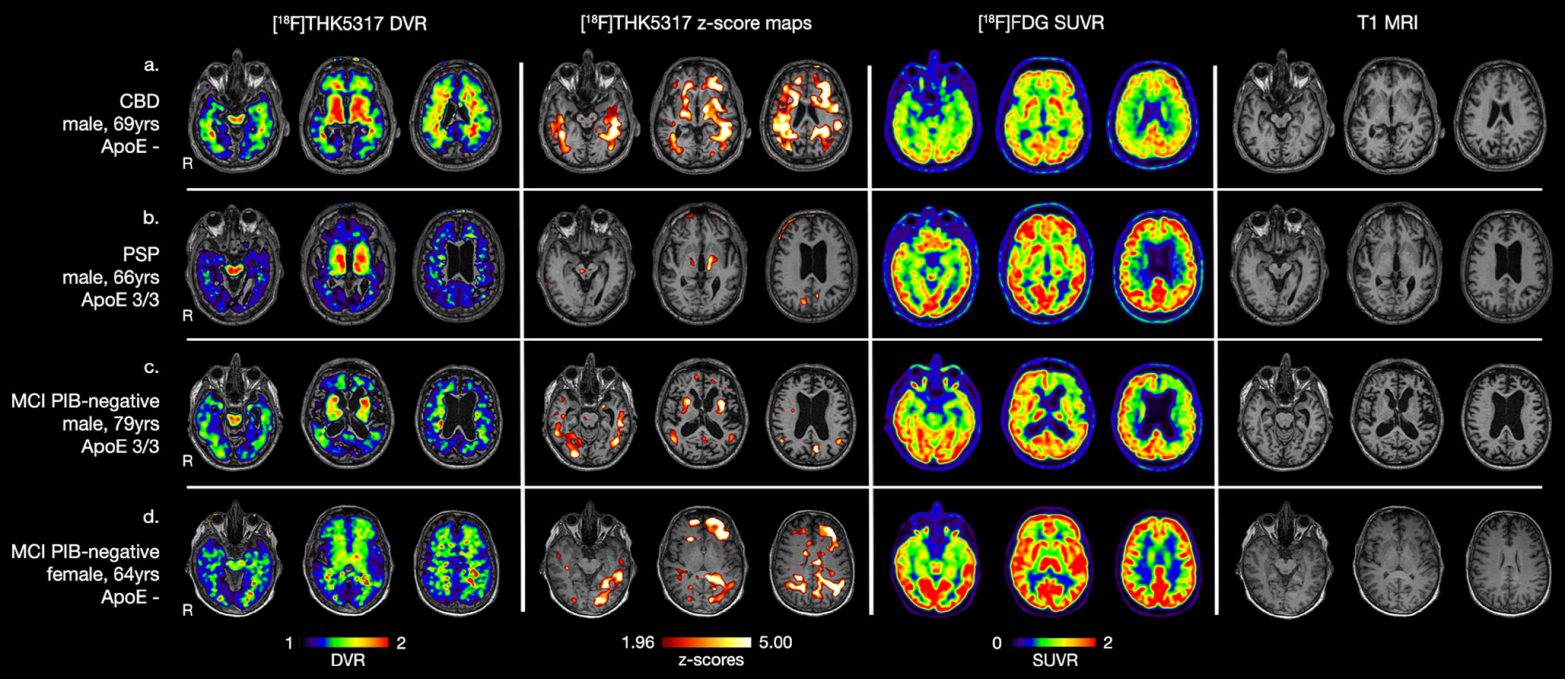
[^18^F]THK5317 DVR retention, [^18^F]THK5317 DVR z-score maps in comparison to young healthy controls (left two columns), [^18^F]FDG SUVR uptake (third column) and T1 MRI (fourth column) in the patients with non-Alzheimer’s disease dementia (first two rows) and the MCI PIB-negative patients (last two rows). A z-score threshold of 1.96 (95 % confidence interval) was applied. CBD = corticobasal degeneration; DVR = distribution volume ratio; MCI PIB-negative = mild cognitive impairment (MCI) with [^11^C]PIB uptake below the normal range (threshold standard uptake value ratio of 1.41); PIB = [^11^C]PIB; PSP = progressive supranuclear palsy; R = Right; SUVR = standard uptake value ratio
